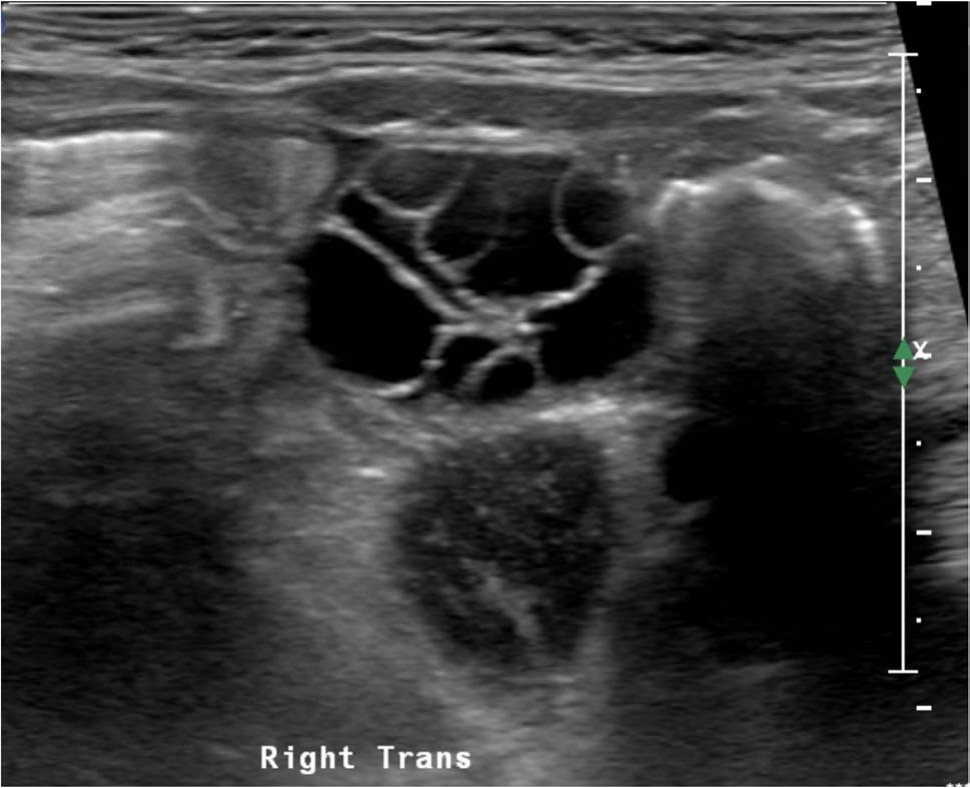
Axial gray-scale pelvic ultrasound image of a normal neonatal ovary, there are multiple follicles of different sizes
